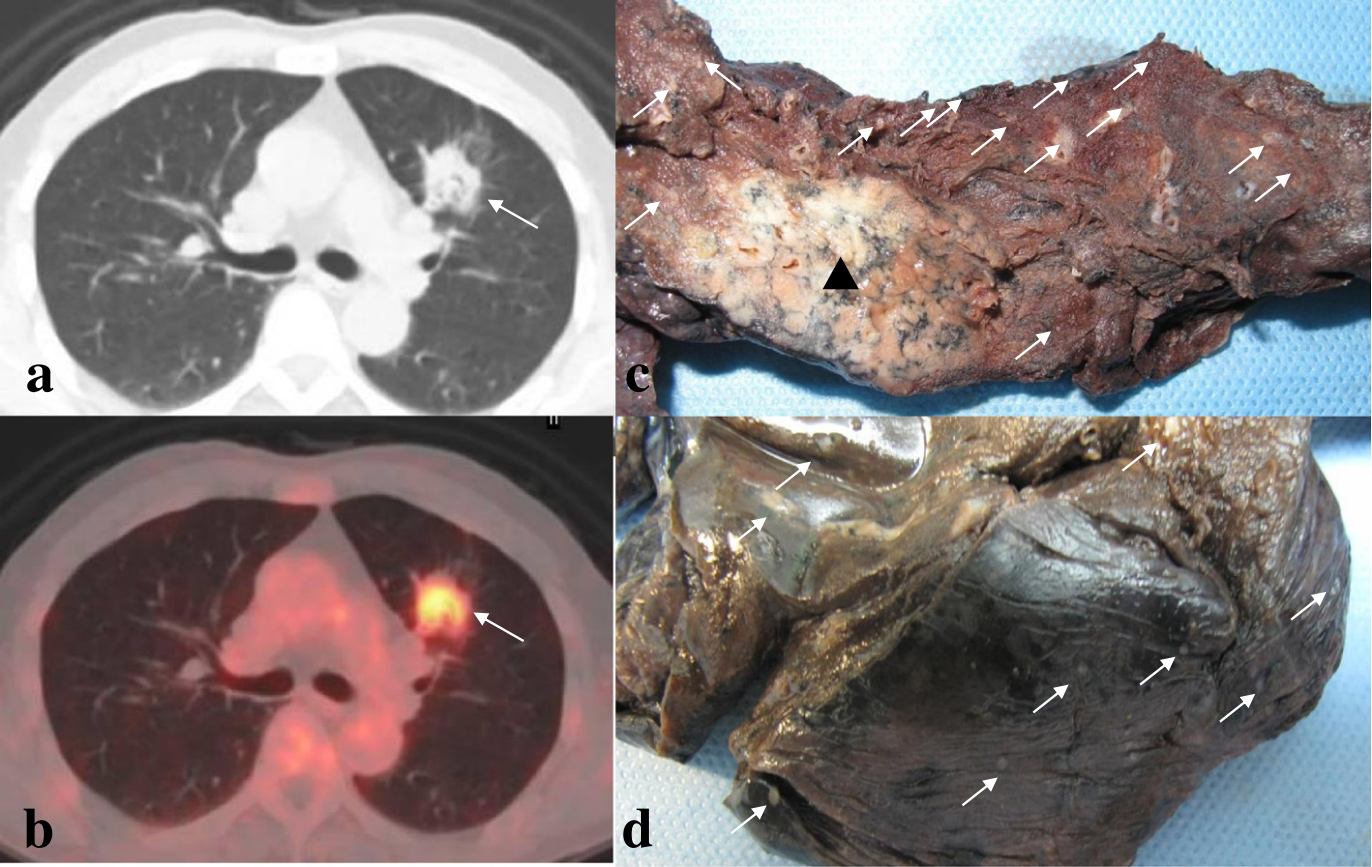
PET-CT imaging features and macroscopic features. CT revealed a large lobular mass in the superior lobe of left lung. The largest dimension of the mass is 3.2 cm. It invaded the anterior segment and superior segment of the left lung, and invaded pleura mediastinalis. The imaging of the rest lung looks normal (a). PET and CT fusion image showed the nodule had intense FDG uptake. The SUVmaxs was 5.2, which suggested a malignant tumor on PET-CT, and probably lung primary cancer (b). Gross examination showed a tumor (▲) in the peripheral of the lung, with the largest diameter of 3.5 cm. The cut section surface of the tumor was hard, grey and fine granular, and there were many small grey nodes (arrows) in the rest lung, with a diameter of 0.1 cm to 0.3 cm (c). There were plenty of small nodes (arrows) on the visceral pleura (d).
